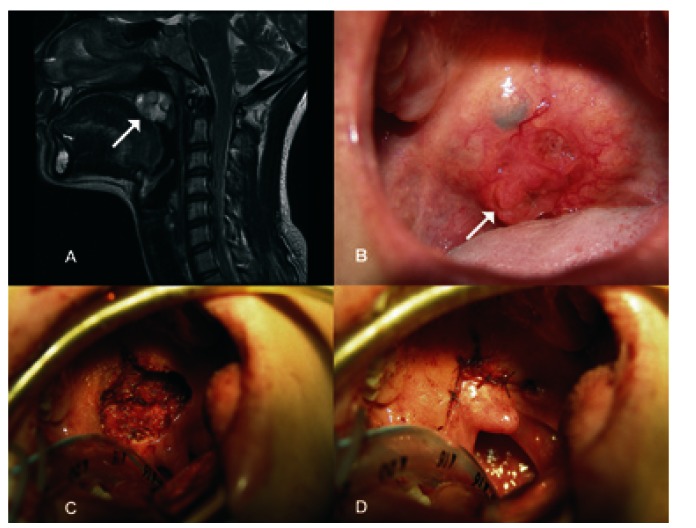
A) Sagittal magnetic resonance imaging (MRI) showing dependent lesions of the soft palate shaping the dome of the tongue at the base. B) Photograph of the lesion showing ulceration. C) Surgical bed after resection with CO2 laser. D) Primary closure of the palate defect.
